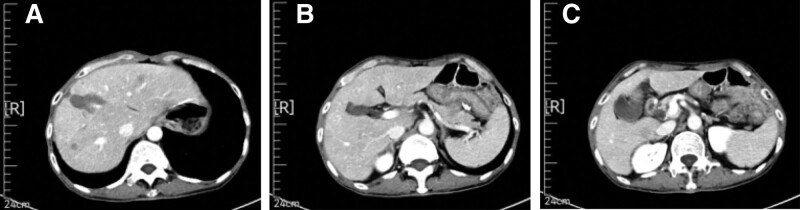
CT (2022-9-14) shows larger nodules in the S6 and S7 segments of the liver compared to previous CT images on June 18, 2022, and nodules in the anterior end of the pancreatic tail. Metastasis is considered. CT = computer tomography.
